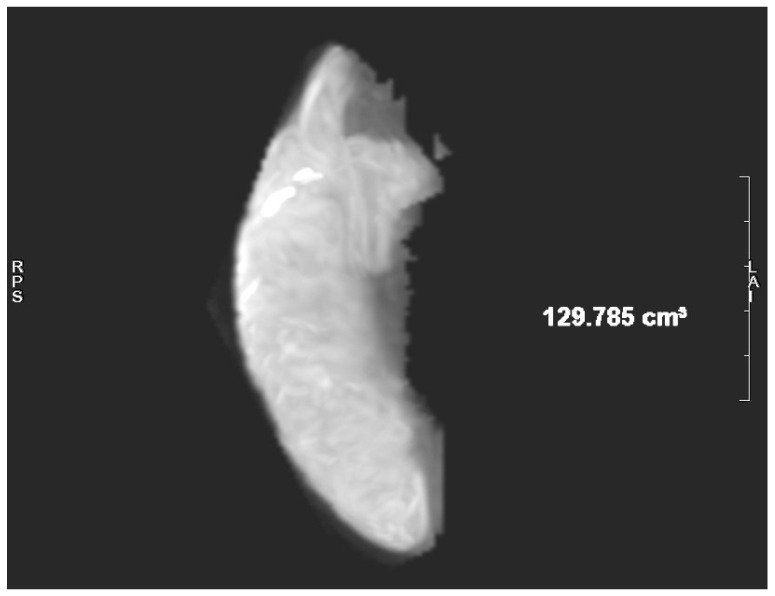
Acute infarction cite after 3 h and its volume on DWI sequence—129,785 cm^3^ (“RPS” right-posterior-superior, “LA” left-anterior).
